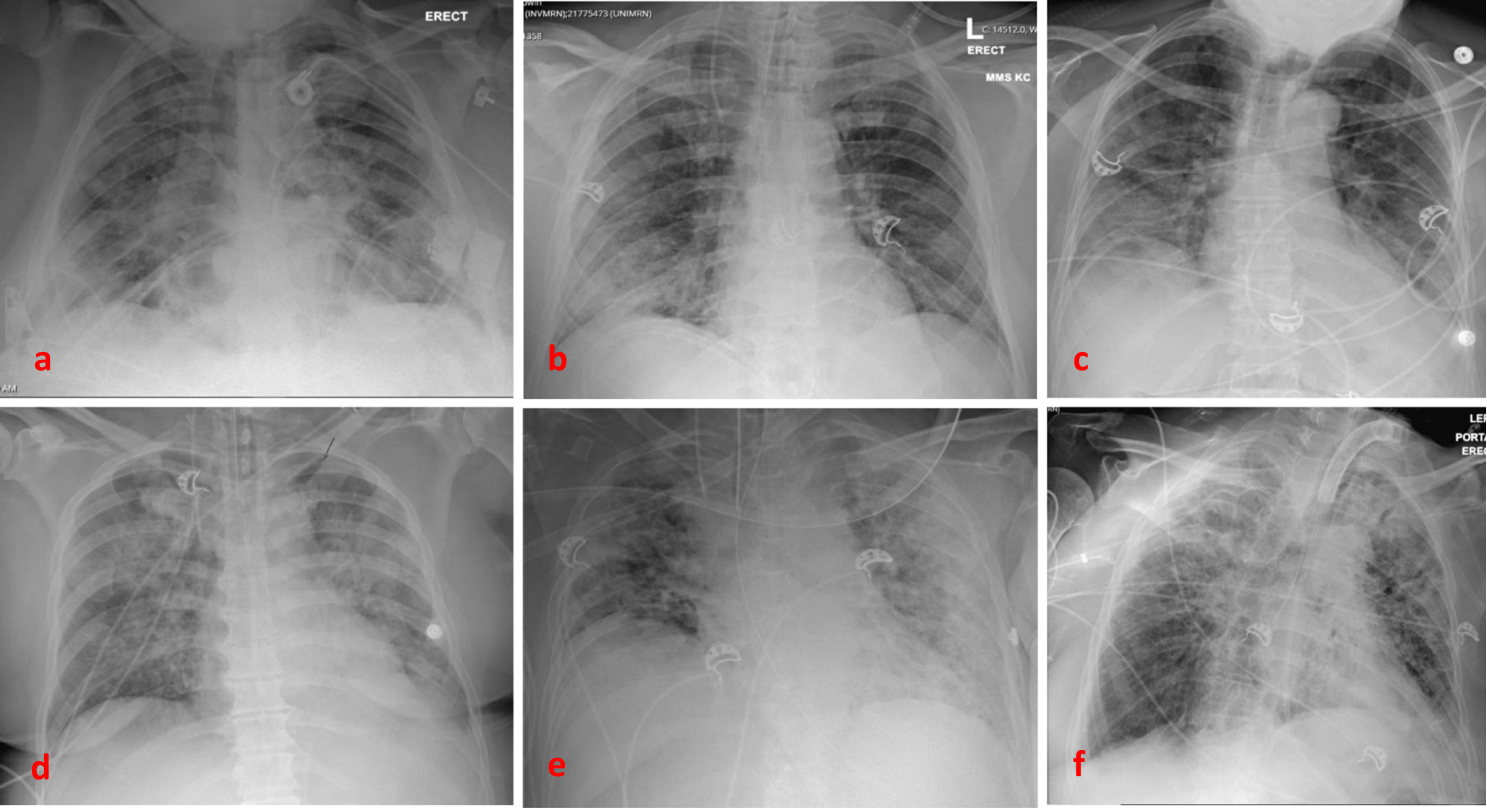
Interim chest radiographs for each patient prior to developing pneumothorax. From top left: a Case-1. b Case − 2. c Case − 3. d Case-4. e Case-5. f Case 6
